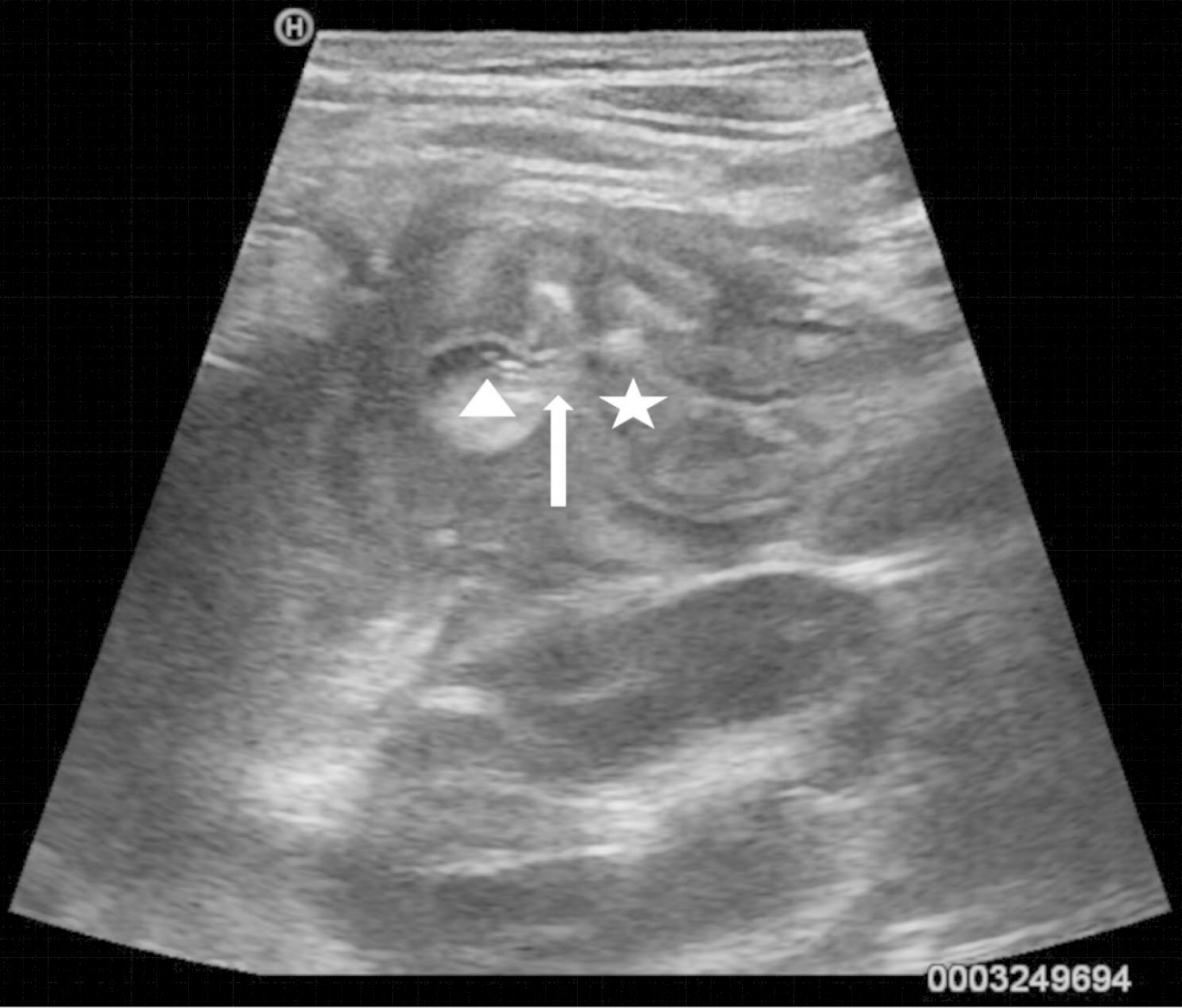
Sonogram of duodenal ulcer perforation. Sonogram shows gas and fluid accumulation around the duodenum(triangle), discontinuous duodenal intestinal wall(arrow). The star mark represents the duodenal cavity
